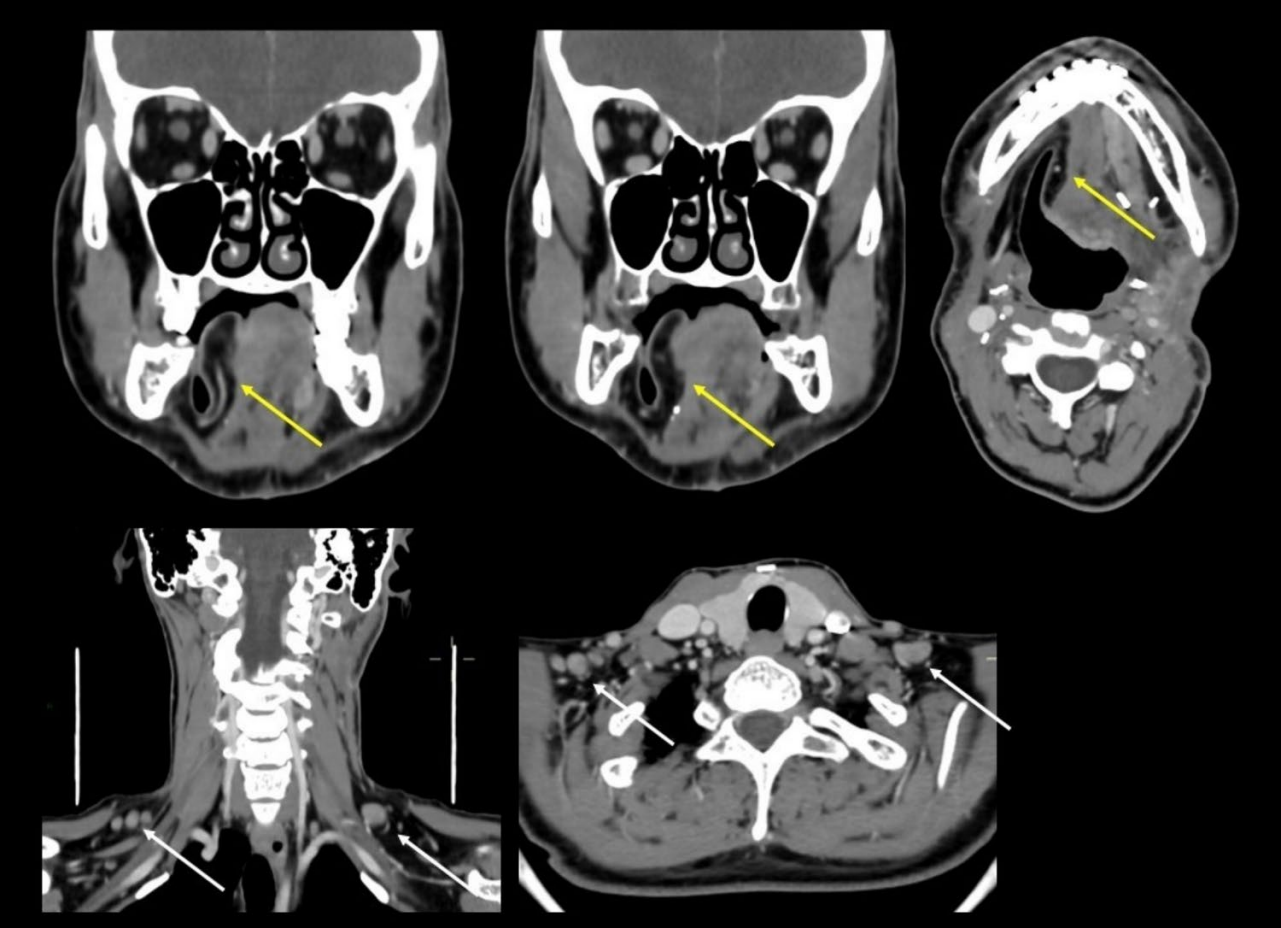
Coronal and axial post-operative contrast-enhanced CT (CE–CT) obtained 4 weeks after surgery in a patient with pT3N2c floor-of-mouth squamous cell carcinoma treated with hemiglossectomy, neck dissection, and radial forearm flap reconstruction. NI-RADS T1: no abnormal enhancement along the superficial or deep margins of the flap (yellow arrows, upper-row images). NI-RADS N2: bilaterally enlarged level Vb lymph nodes without necrosis or signs of extranodal extension (white arrows, lower-row images)
